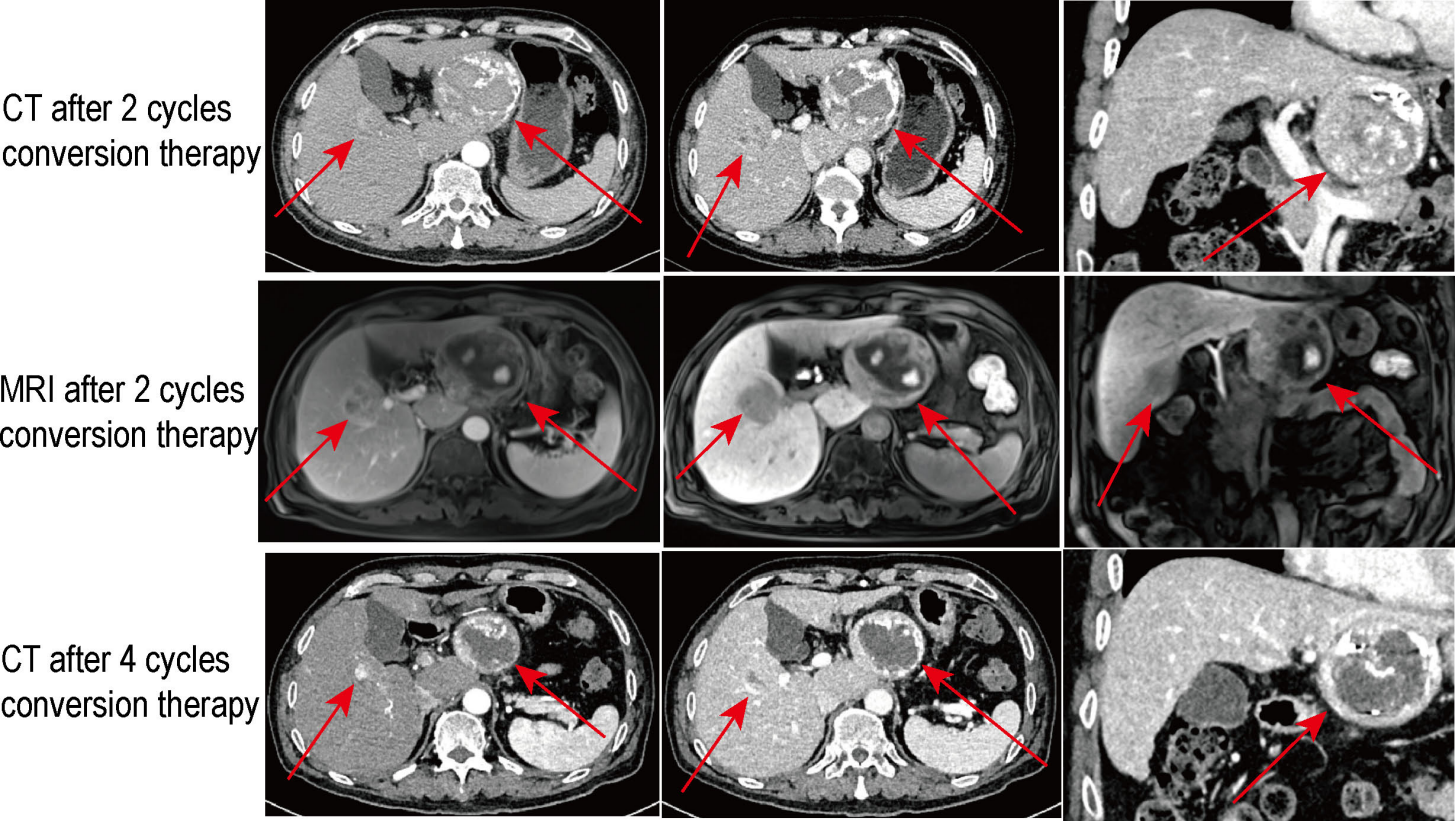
The multiphase CT and gadoxetate disodium–enhanced MRI scan results for review after the conversion therapies of various periods. The red arrows indicate the intrahepatic bilobar tumors.
